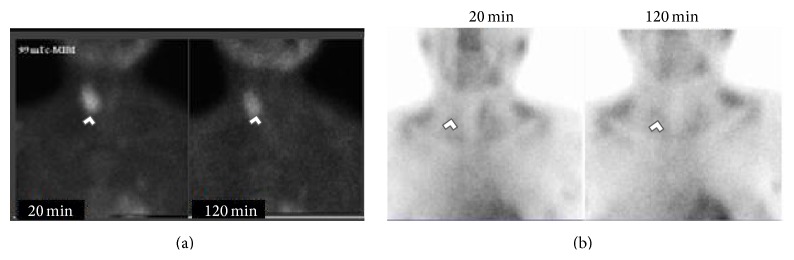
Neck scintigraphies with 25 mCi of ^99^Tc-sestamibi with 0′ and 120′ wash-out sequences. (a) 2010 Admission Scintigraphy. 120′ washout sequence shows residual capitation from right lower thyroid lobe suggesting a parathyroid adenoma. (b) 2013 postparathyroidectomy control scintigraphy. 120′ wash-out sequence shows no apparent residual captation. Findings are marked with white arrow heads.
